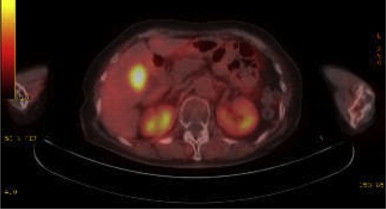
A follow-up PET–CT revealed the uptake of FDG only at the gallbladder (SUV_max_ 7.1).
